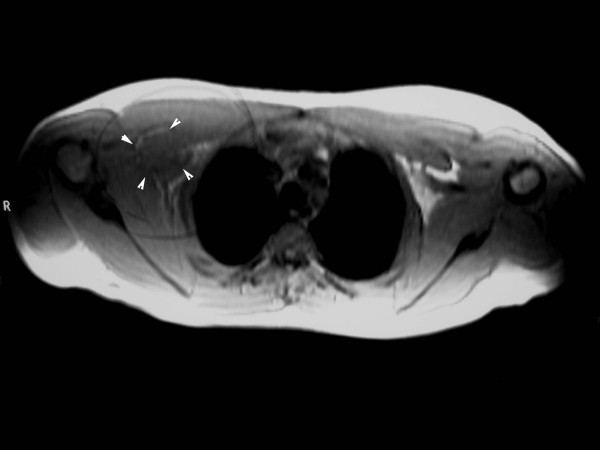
T1-weighted magnetic resonance imaging scan. A large mass (white arrowheads) that was suspected to be a hematoma was present, compressing the brachial plexus in a space between the subscapular and pectoralis minor muscles.
